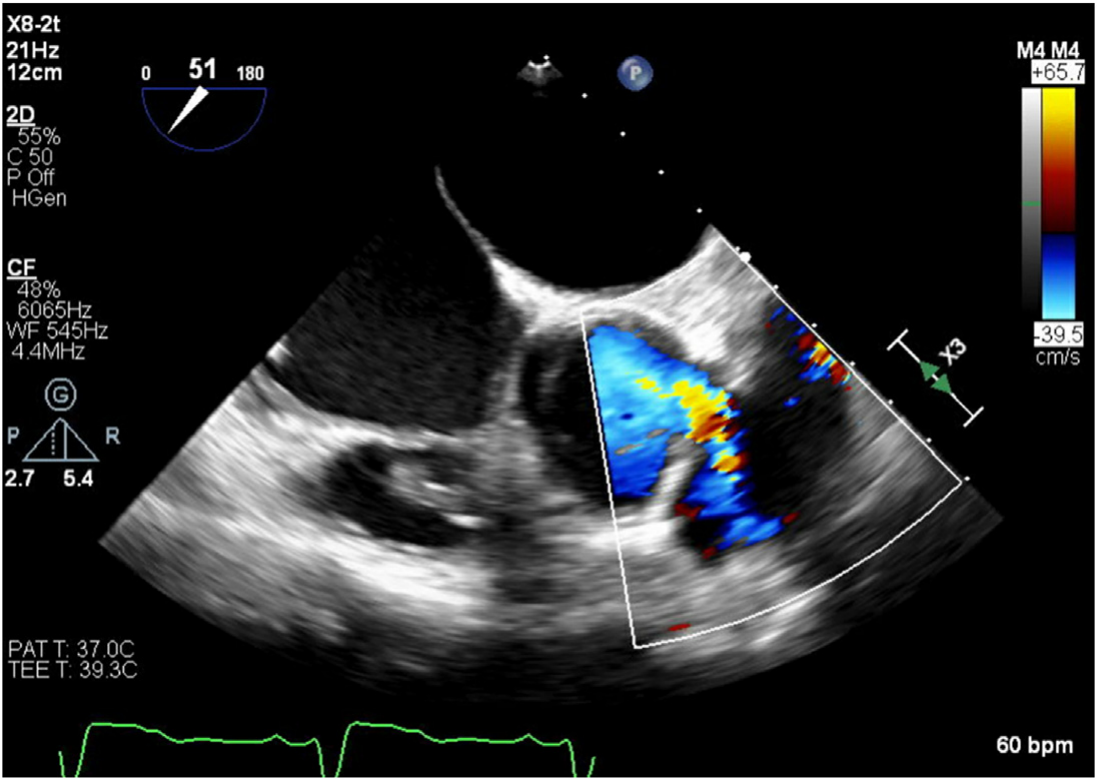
Transesophageal echocardiogram of the midesophageal right ventricle inflow-outflow window with color Doppler. Demonstrated is an aortopulmonary fistula near the left coronary cusp to the pulmonary artery with a left-to-right shunt.
